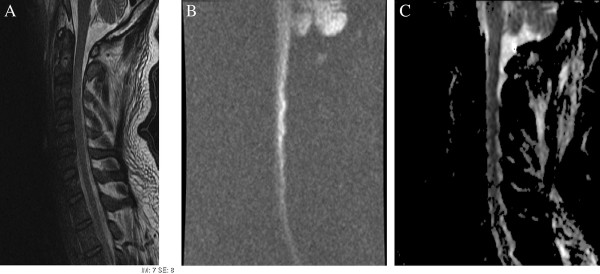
Magnetic resonance imaging of the cervical spine repeated eight days after the initial magnetic resonance image performed on admission. Interval development of cord edema and infarction is seen from the C2 to T2 levels. (A) Sagittal T2 sequence. (B) Diffusion-weighted image. (C) Apparent diffusion coefficient image. The diffusion-weighted image and apparent diffusion coefficient image do not demonstrate the exact same level of involvement, with the apparent diffusion coefficient image showing signal changes slightly lower in the cervical spine than the diffusion-weighted image. Also, the diffusion-weighted image signal abnormality does affect somewhat the dorsal aspect of the cord. This reflects the often diffuse and collateral nature of electrical injuries.
